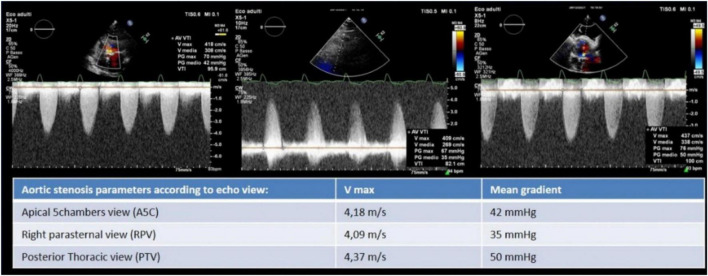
Aortic stenosis severity evaluation by Vmax and mean gradient from apical five chambers, right parasternal, and posterior thoracic view where the latter allowed to record the highest Vmax and mean gradient.
